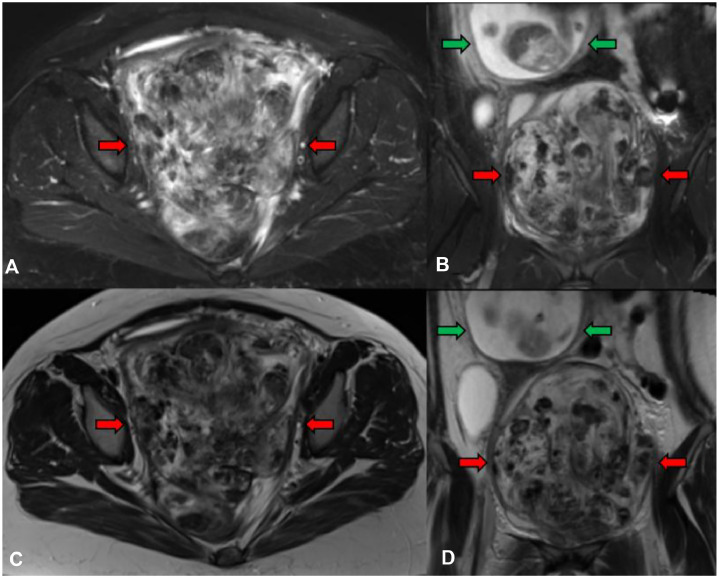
Initial MRI of the pelvic AAM (continued). Axial and coronal T2-weighted images demonstrated mixed areas of hypo- and hyperintense signals throughout the mass (red arrows). There was no significant signal dropout on the fat suppressed images (A, B) compared to the nonsuppressed images (C, D) to suggest the presence of macroscopic fat. The gravid uterus (green arrows) was displaced superiorly to the right of midline.
